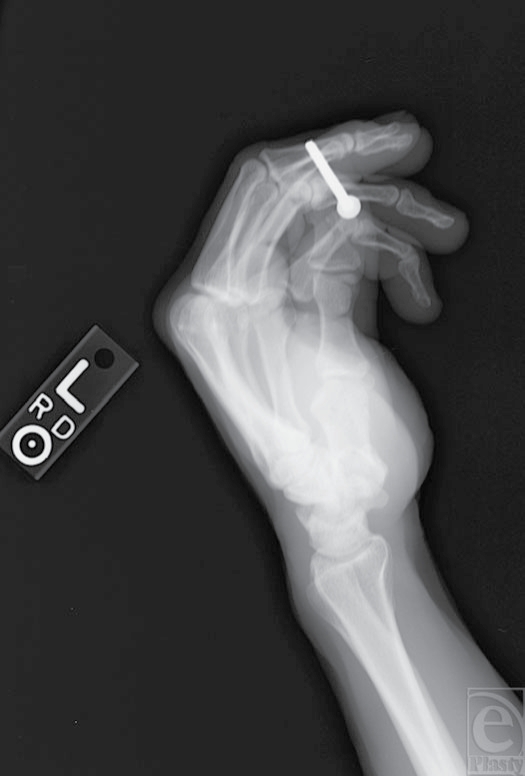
X-ray film demonstrates no bony injury to phalanges (oblique view).
